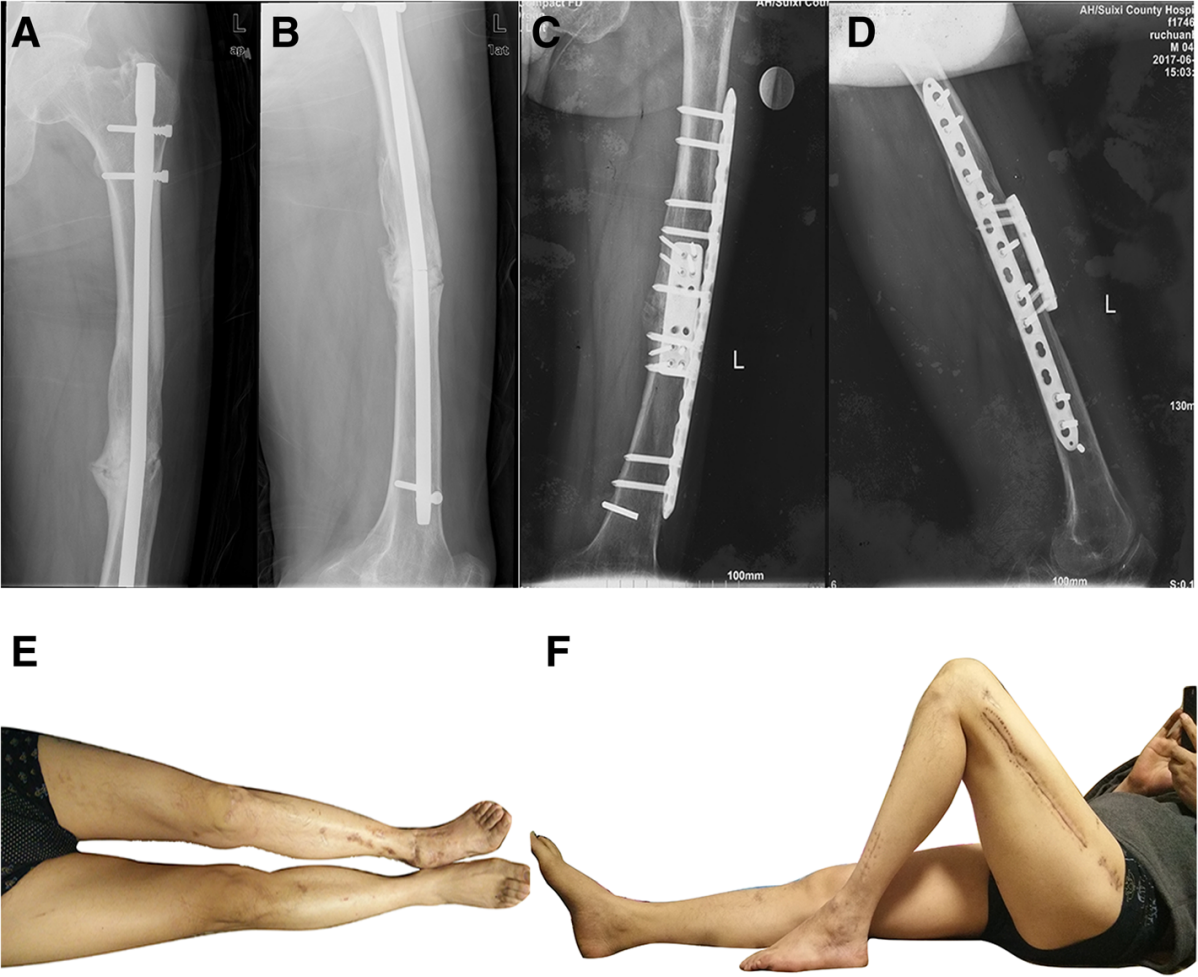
A 43-year-old male with left femoral shaft non-union after nailing. a, b X-ray showing breakage of the intramedullary nail and distal locking screw with obvious internal rotation and varus deformity preoperatively. c, d X-ray showing fracture healing 7 months after DP. e, f The knee joint function of the affected side was good
